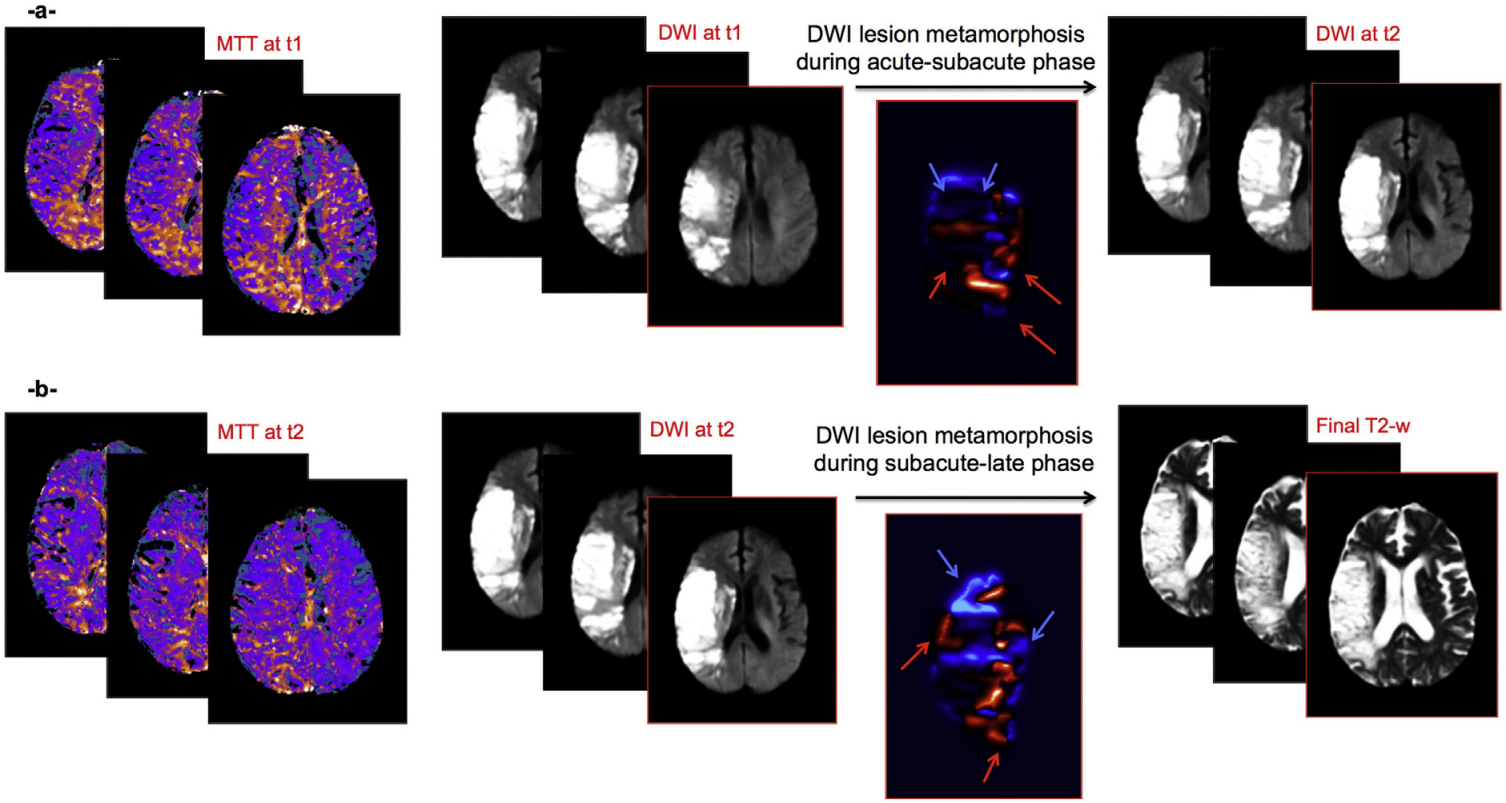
Phase-based DWI lesion metamorphosis in light of spatiotemporal perfusion values. (a) Acute–subacute phase data: Axial images of acute MTT (left), acute DWI (middle) and subacute DWI (right). (b) Subacute-final phase data: Axial images of subacute MTT image (left), subacute DWI image (middle) and final T2-w image (right). During acute–subacute phase, we metamorphose acute DWI lesion to subacute DWI lesion. During subacute-final phase, we deform the latter into final T2-w lesion. We estimate the deformation maps for both phases of DWI lesion evolution (images under the black arrows). The red arrows point to highly expanding (red) areas and blue arrows point to highly contracting (blue) areas.
